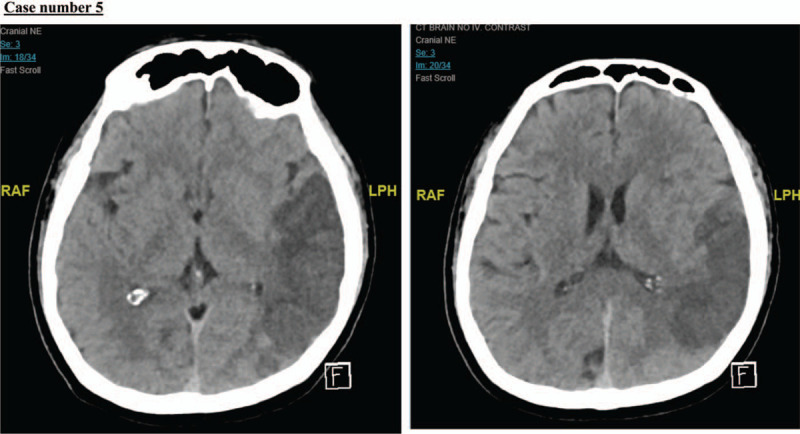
Plain computed tomography (CT) brain showed a major left middle cerebral artery (MCA), and posterior cerebral artery territory infarction with effacement of the cortical sulci.
